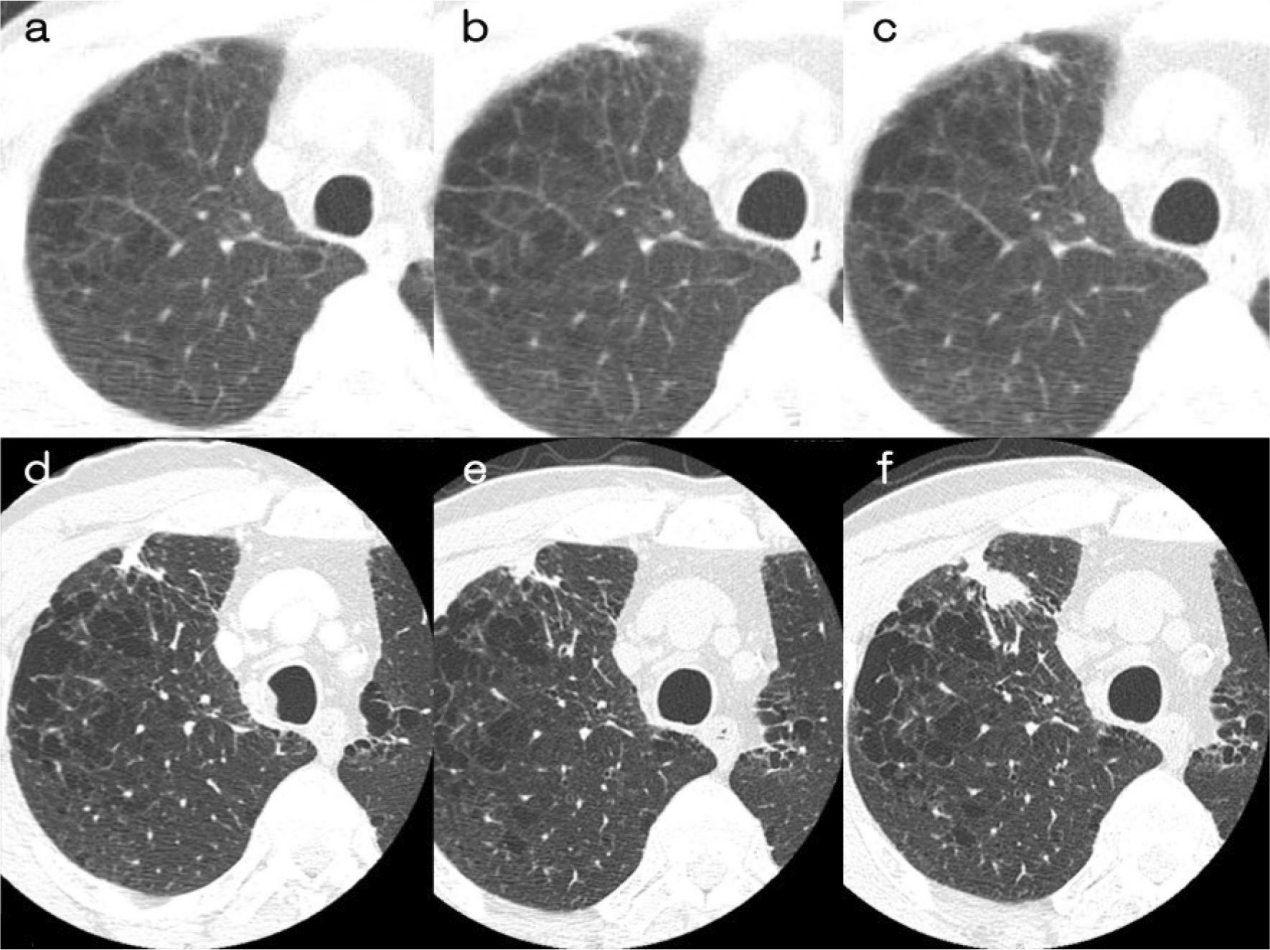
Band opacity appearing in emphysema. a A screening CT image taken 2 years prior to referral to a medical institution. Moderate emphysematous changes are observed in the background lung, and a band opacity is noted near the pleura in right S3b. b One year before the referral, the screening CT shows an increase in the attenuation value of the band opacity. c At the time of referral to the medical institution, the width of the opacity in the dorsoventral direction had increased. d a A thin-section CT image taken at the medical institution. e Two months later, a follow-up thin-section CT image shows no temporal increase in the band opacity. f Six months later, a significant increase in the proximal portion of the band opacity is observed. It was diagnosed as squamous cell carcinoma, cT2aN2M0. It should be noted that lung cancer does not always manifest as a typical pulmonary nodule morphology
